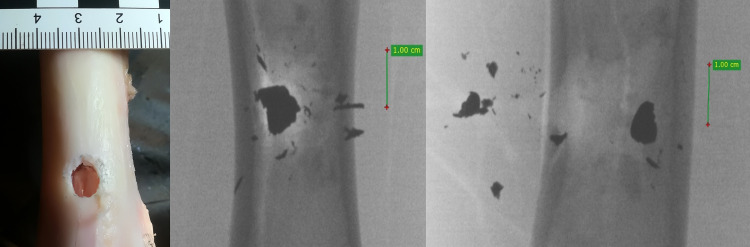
H&N Baracuda Hunter airgun pellet cal. 6.35 mm, from the left: entry wound damage, A-P X-ray, lateral X-ray. Shot to the anterior surface of the femoral shaft.
